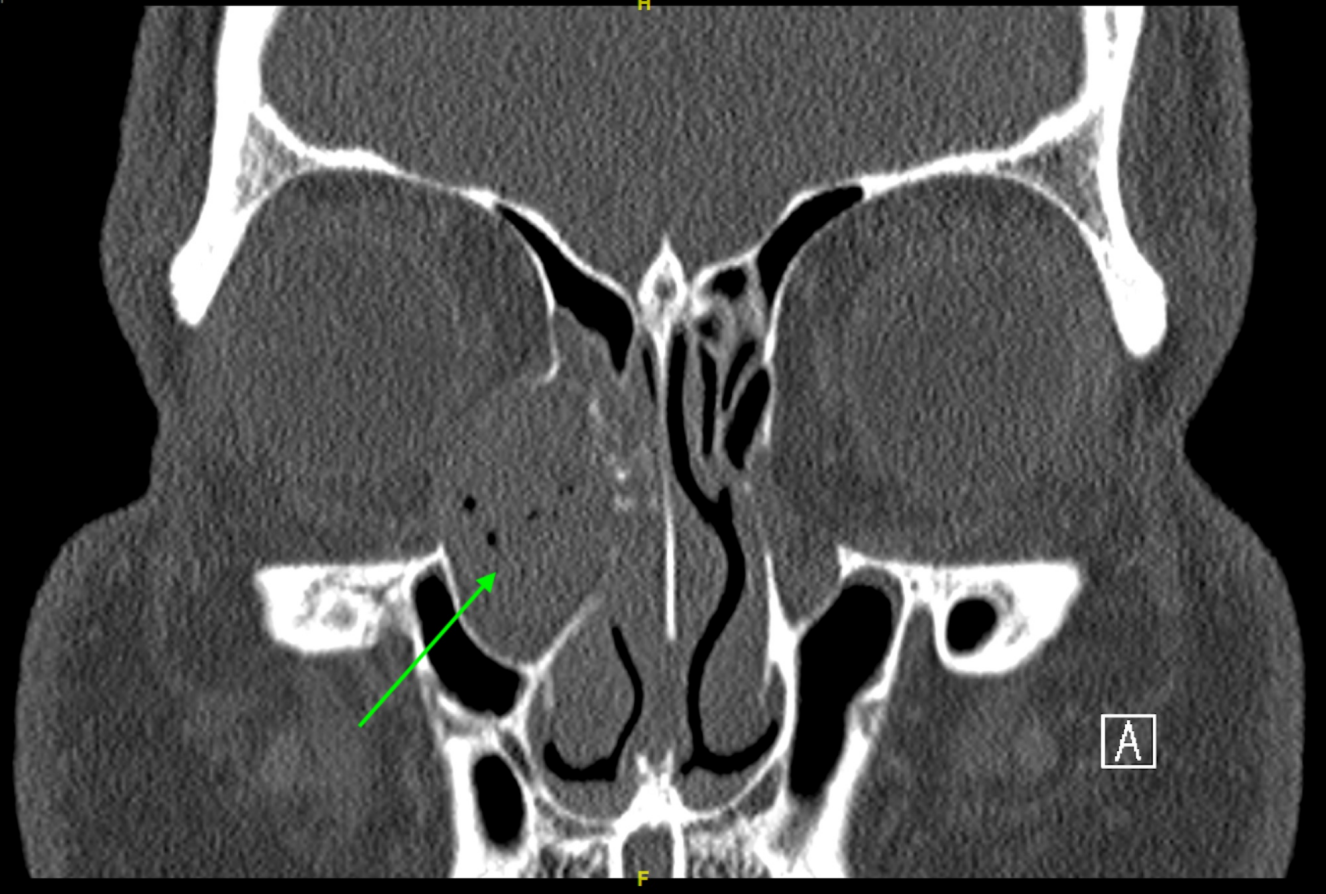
CT scan of orbits showing a 2.6 cm lesion centered upon the right nasolacrimal duct with adjacent bony remodeling and expansion, as well as partial dehiscence of the medial orbital wall.
